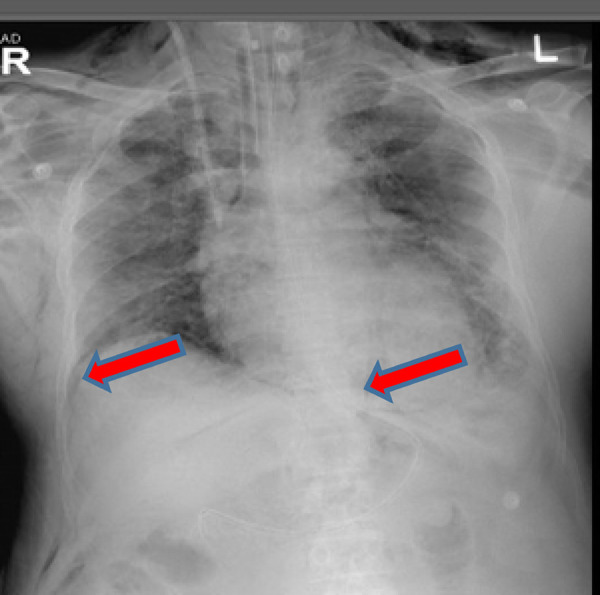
Chest X-ray image showing pneumomediastinum
